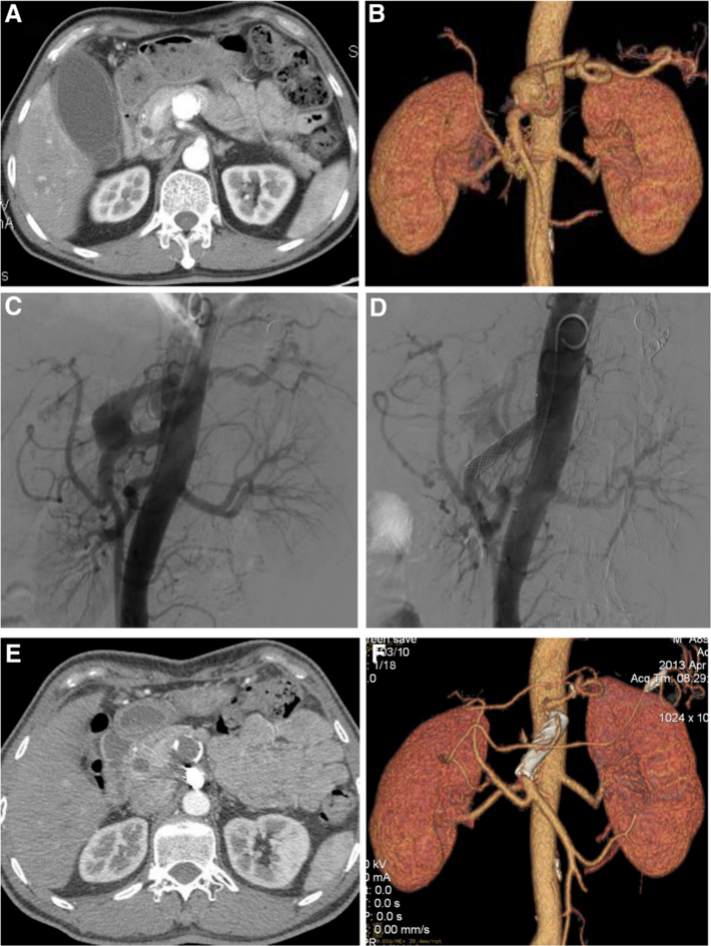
The image finding of case three during preoperation, intraoperation and follow-up. A, B and C, Preoperative CTA and DSA demonstrated a 35 mm × 34 mm SAA near the origin of the SMA and the proximal neck of the SAA was 5 mm. D, The completion aortogram revealed evidence of a small endoleak; disappearance of the aberrant splenic artery aneurysms lumen and patency of the SMA. E and F, The CTA in 10 months postoperatively demonstrated that the SAA sac was thrombosed with marked shrinkage; the SMA is patent with no infarction or abscess formation in the spleen.
